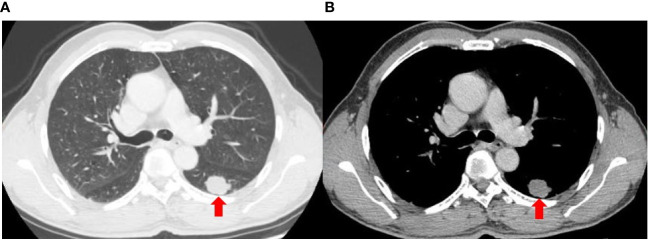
Axial computed tomography images of the lung (A) and mediastinal (B) window show a lobular soft tissue mass in the left lower lobe.
